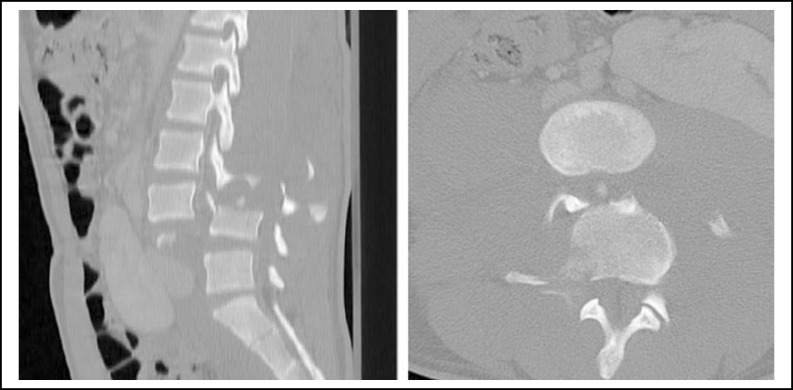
CT scan showing complete dislocation in the L3 – L4 in the axial view and its Sagittal restoration
